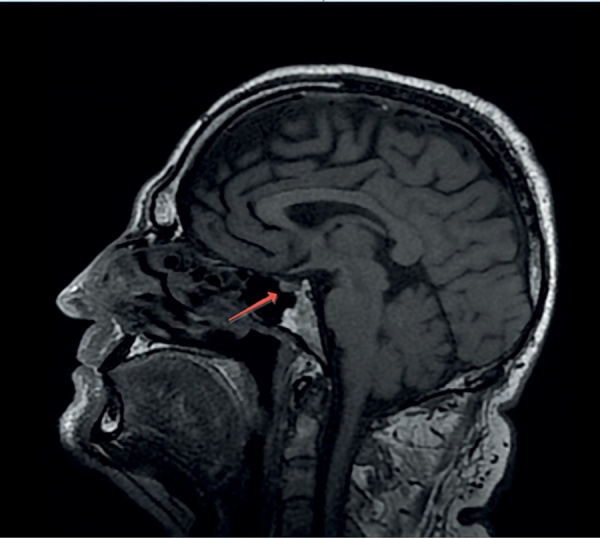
Рисунок 2. МР-картина хиазмально-селлярной области пациента. Сагиттальный срез.Figure 2. MRI of the patient's chiasmal-sellar area. Sagittal section.
